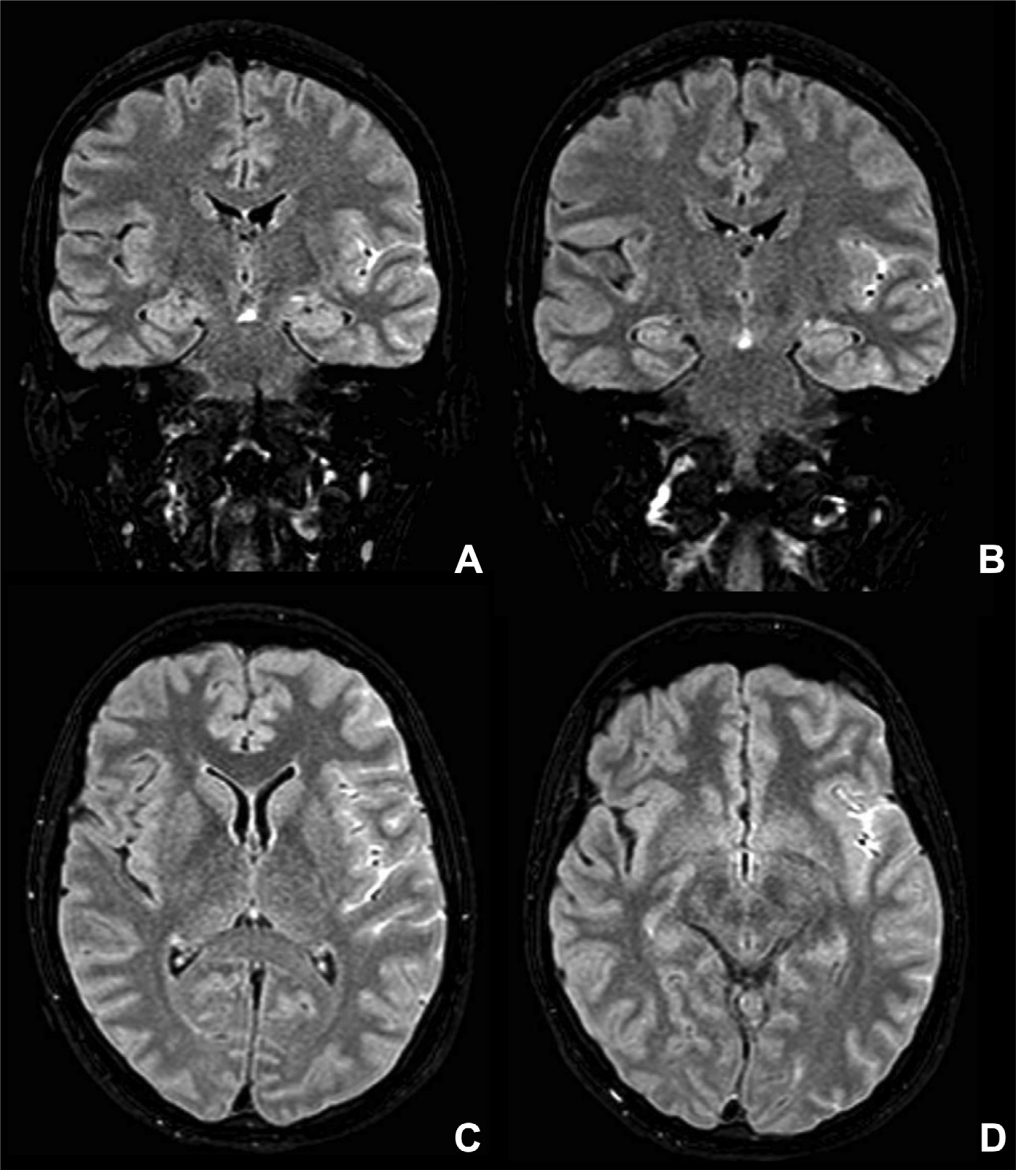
Magnetic Resonance Imaging (MRI) brain findings The panel depicts the patient’s MRI scans taken (performed in the acute phase two days after the admission). A) and B) coronal Fluid-attenuated Inversion Recovery (FLAIR) T2-weighted images, C) and D) axial FLAIR T2-weighted images show hyperintense alterations in the left temporo-fronto-parietal cortex.
